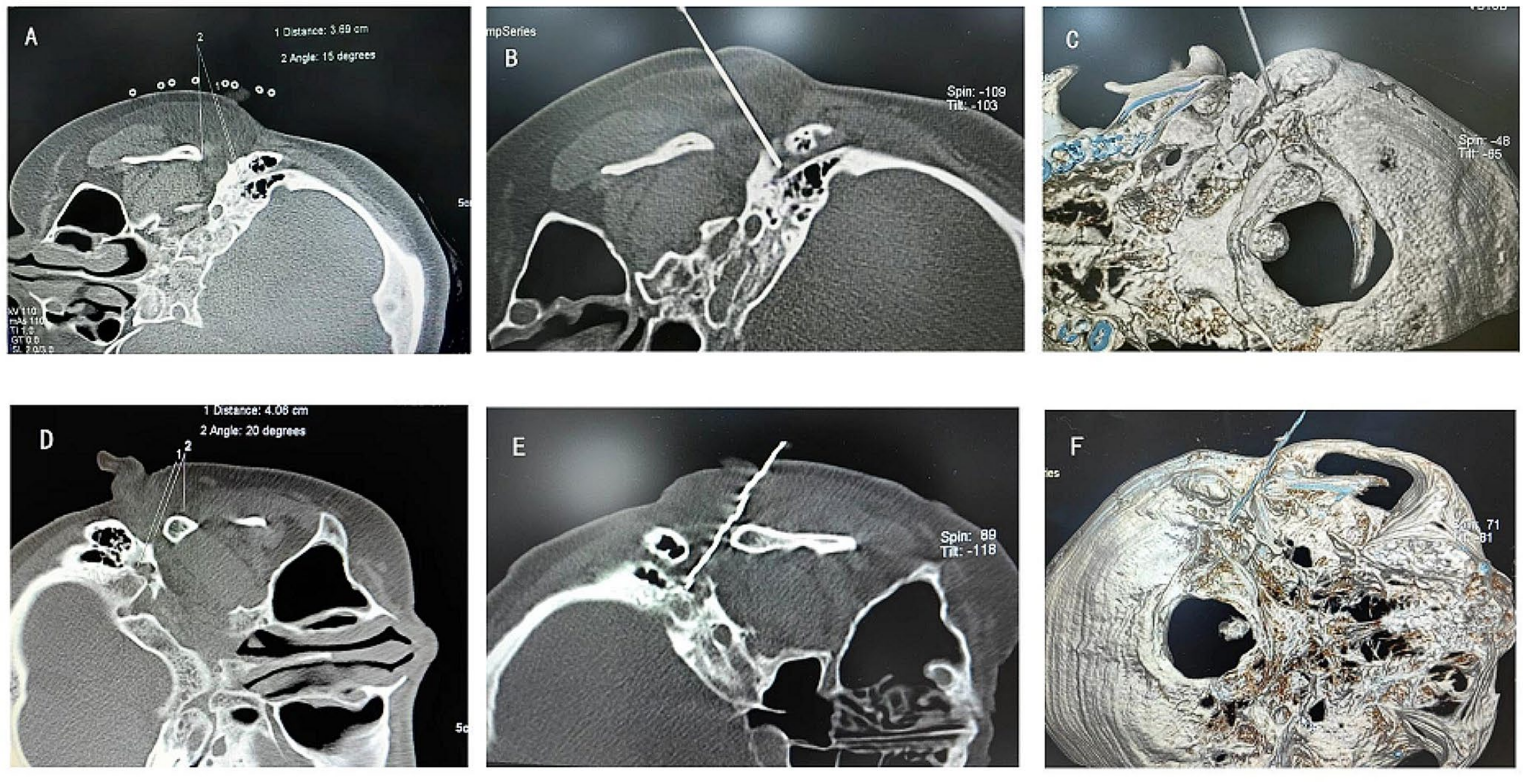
CT-guided radiofrequency ablation of facial nerve by bilateral stylomastoid foramen puncture. (A) Right stylomastoid foramen puncture path design and puncture parameters (depth, angle) measurement; (B) Right stylomastoid foramen puncture was successful; (C) CT three-dimensional reconstruction image after successful right stylomastoid foramen puncture; D, E and F are the left side
